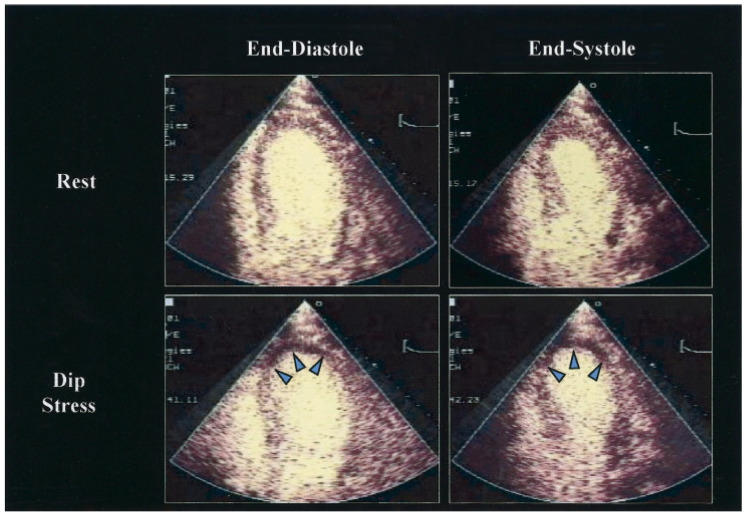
Dipyridamole (Dip) stress contrasts echocardiography using real-time perfusion imaging at a low mechanical index, showing images at end diastole and end systole in a patient with significant stenosis of the left anterior descending coronary artery. Note the perfusion defect that developed in the apex (highlighted by arrows) and the corresponding wall motion abnormality. Reprinted from Journal of the American College of Cardiology, Volume 45, Issue 11, Armstrong, W.F. et al. [122], Stress Echocardiography: Current Methodology and Clinical Applications, pages 1739–1747, Copyright 2005, with permission from Elsevier.
